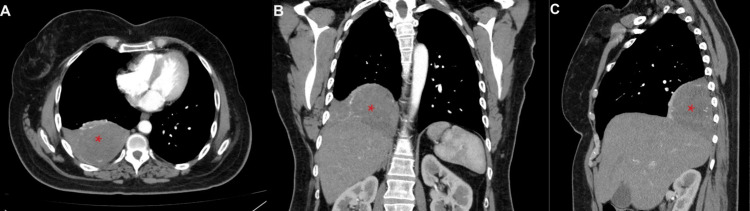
Contrast-enhanced CT scan of the chest revealing a large right-sided pleural solid vascular mass consistent with a solitary fibrous tumor. Combined axial (A), coronal (B), and sagittal (C) views of the CT scan of the chest with contrast. A large right-sided pleural solid vascular mass measuring 10x9x6 cm is visualized (asterisk), along with passive atelectasis in the base of the right lung, left apical fibrotic changes consistent with postradiation fibrosis, and left adrenal thickening.
